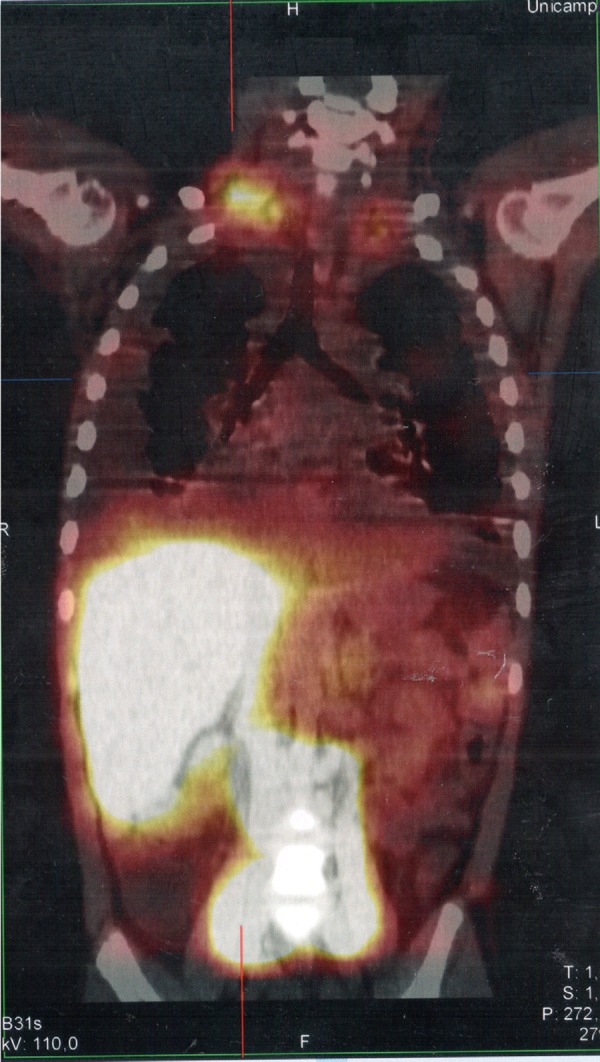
SPECT/CT image of the cervicothoracic and abdominal regions obtained from the lymphoscintigraphy assessment with intradermal administration of dextran-99mTc on the dorsum of the feet; the images obtained 18hours after start of the examination show focal area of radiotracer retention/leakage in the lymph ducts located in the topography of the thoracic introit, bilaterally, more accentuated to the right.
